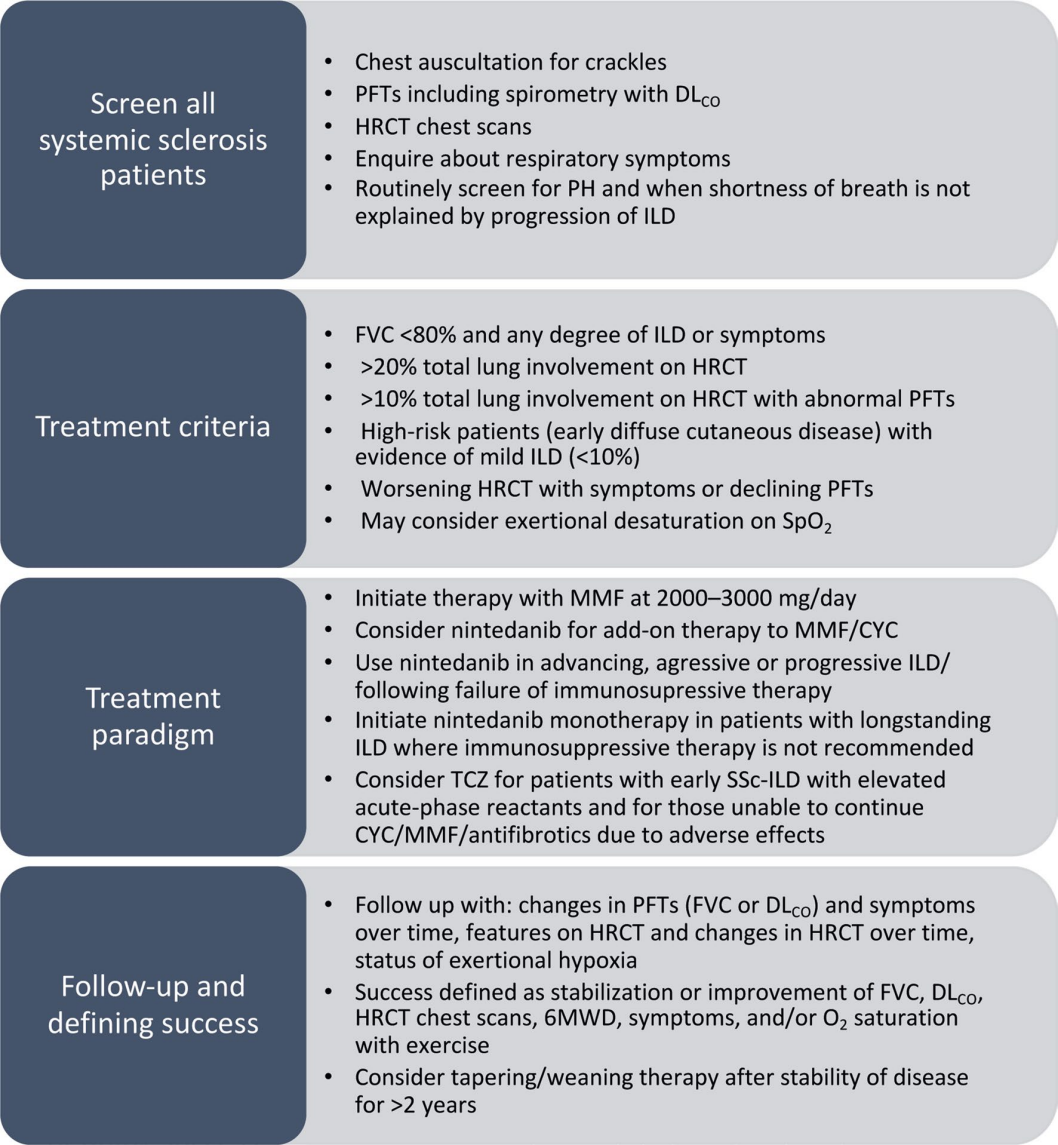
Summary of the consensus recommendations for the management of patients with SSc-ILD. *6MWD* 6-min walk distance, *CYC* cyclophosphamide, *DL*_*CO*_ diffusing capacity of the lungs for carbon monoxide, *FVC* forced vital capacity, *HRCT* high-resolution computed tomography, *ILD* interstitial lung disease, *MMF* mycophenolate mofetil, *PFT* pulmonary function test, *PH* pulmonary hypertension, *SpO*_*2*_ peripheral capillary oxygen saturation, *SSc-ILD* systemic sclerosis-associated ILD, *TCZ* tocilizumab
